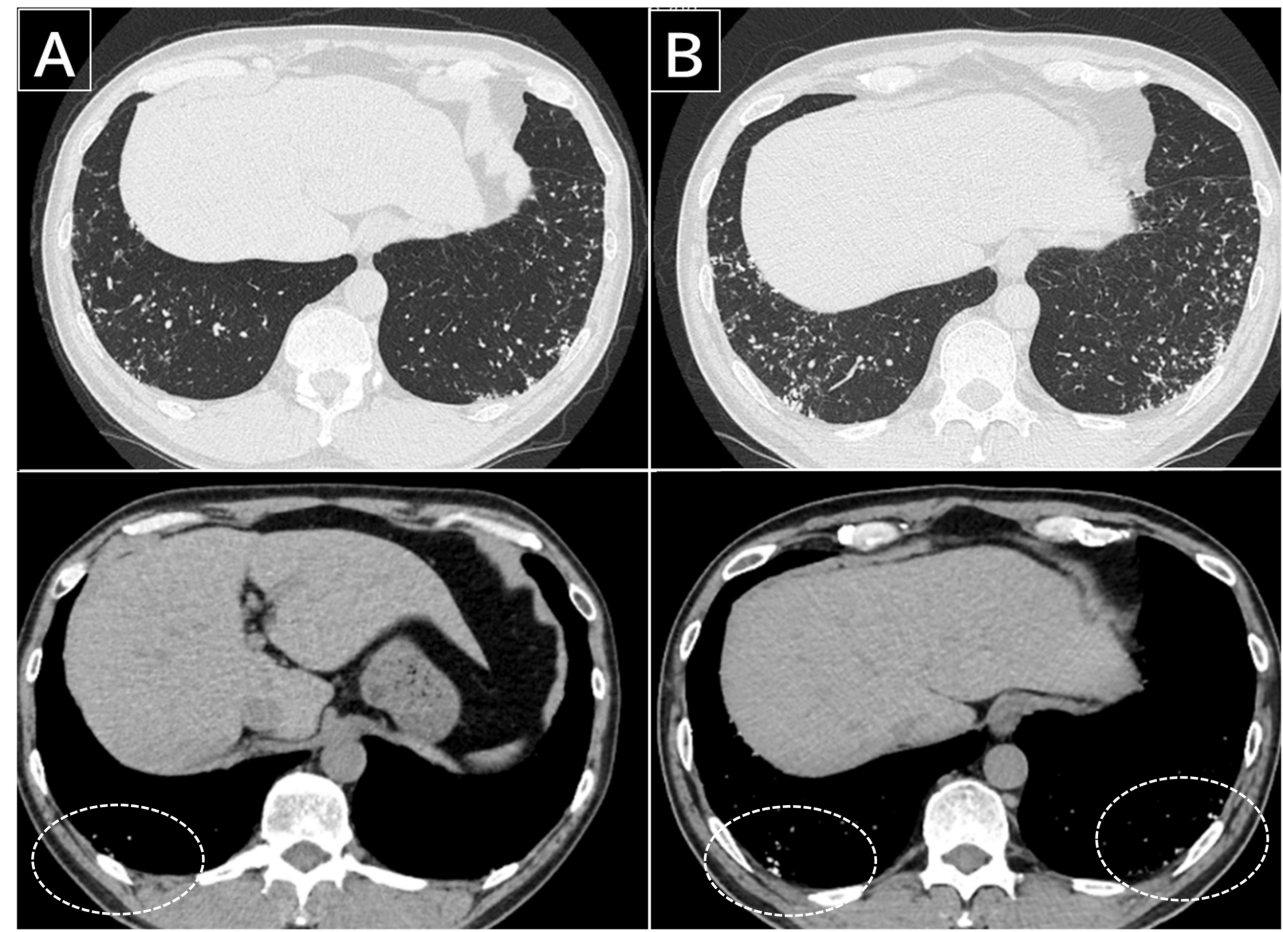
Chest computed tomography findings over time (A) Bilateral, diffuse, linear, and branching structures with calcification predominantly in the lower lung lobes (circle). (B) Progression of ossified lesions over a five-year period.
